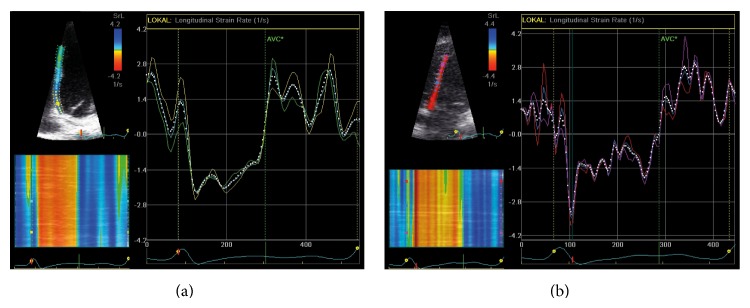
Speckle-tracking*. Longitudinal* strain rate* at maximal stress during exercise echocardiography (a) in a hypertensive patient and (b) in a healthy control. Note the difference in maximal* strain rate* during early systole.
